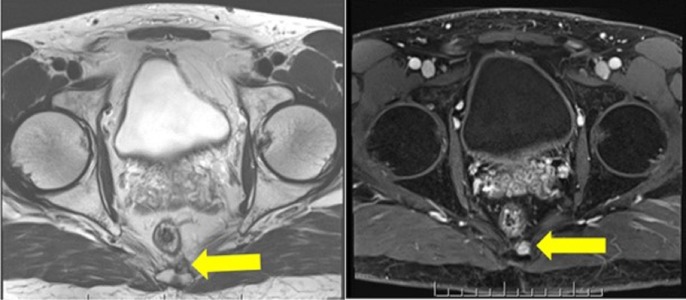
Axial T2-weighted (left) and DCE MRI (right) sequences showing a nodule located left and anterior to the lower coccygeal segment, which demonstrates intermediate hypointensity in T2 and DCE abnormal enhancement DCE: dynamic contrast-enhanced; MRI: magnetic resonance imaging.
